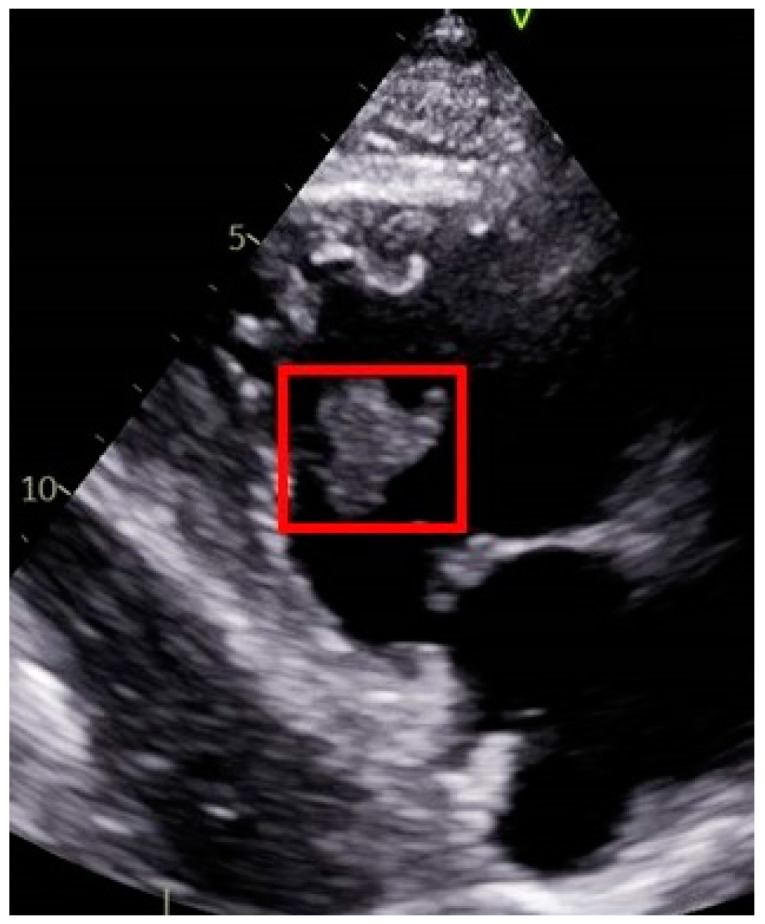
Images from the patient’s echocardiograph showing a decreased diameter of the cardiac mass from the previous scan (red square).
